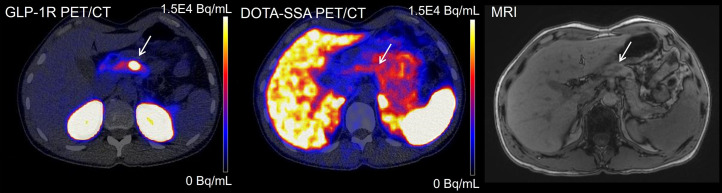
Images of exendin PET/CT, DOTA-SSA-PET/CT, and CE-DWI-MRI (T1-weighted precontrast) of patient 8. Location of insulinoma in pancreas body is indicated with arrows. Only exendin PET/CT was true positive in this patient.
